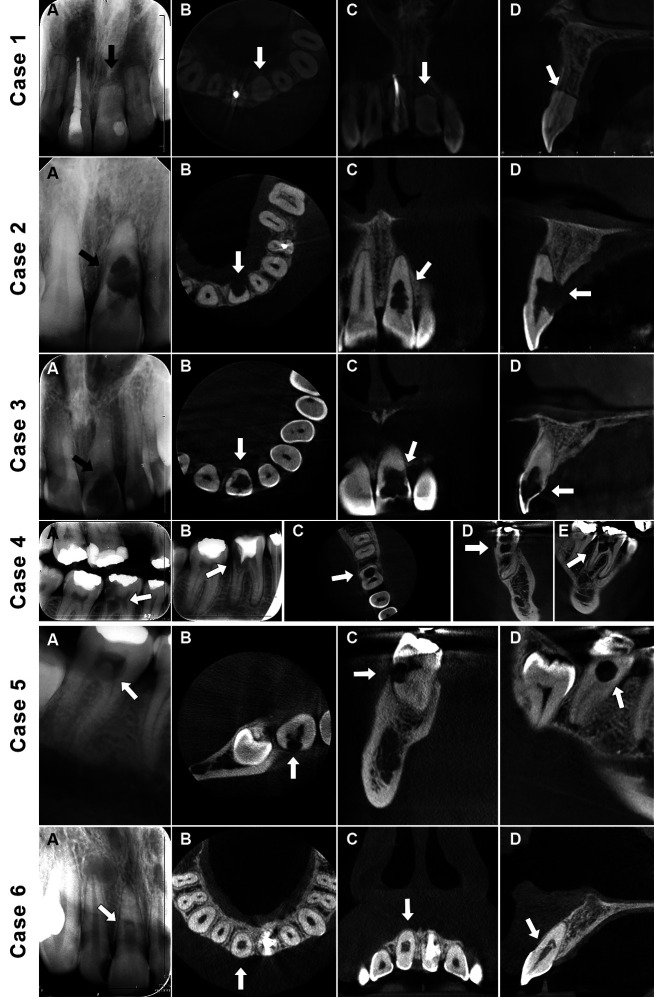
Case 1 (External apical root resorption): (A) A periapical radiograph of maxillary left central incisor. (B, C, D) CBCT reconstructed (axial, coronal, sagittal) views. Case 2 (External inflammatory resorption): (A) A periapical radiograph of maxillary left central incisor. (B, C, D) CBCT reconstructed (axial, coronal, sagittal) views. Case 3 (Internal inflammatory resorption): (A) A periapical radiograph of maxillary left central incisor. (B, C, D) CBCT reconstructed (axial, coronal, sagittal) views. Case 4 (Internal inflammatory resorption): (A) A bitewing radiograph of mandibular right first molar. (B) A periapical radiograph after treatment attempt by a general dentist. (C, D, E) CBCT reconstructed (axial, coronal, sagittal) views. Case 5 (External cervical resorption): (A) A periapical radiograph of mandibular right second molar. (B, C, D) CBCT reconstructed (axial, coronal, sagittal) views. Case 6 (Internal inflammatory resorption): (A) A periapical radiograph of maxillary right central incisor. (B, C, D) CBCT reconstructed (axial, coronal, sagittal) views. Arrows point to the teeth displaying resorption defects.
